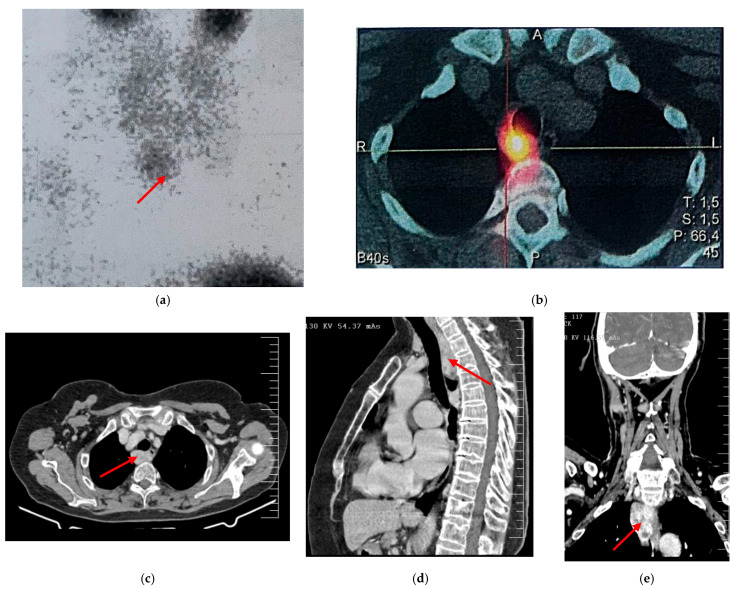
Preoperative imaging of a retrotracheal/paraesophageal adenoma (pointed with a red arrow), including contrast-enhanced CT and sestamibi scintigraphy. Parathyroid scintigraphy (a) performed using the “washout” technique revealed: radiopharmaceutical uptake at the upper mediastinal level. This area persists with increased metabolic activity in the late images. SPECT/CT acquisition (b) highlights an uptake area corresponding to a structure located retrotracheally, right paraesophageal, slightly shifting the esophagus to the left, reaching the level of the D4 vertebral body, measuring 19 mm, suggesting a hyperfunctioning ectopic parathyroid gland. There is no other pathological MIBI uptake at the cervical or ectopic level. CT scans (c–e) show the retrotracheal/paraesophageal adenoma.
